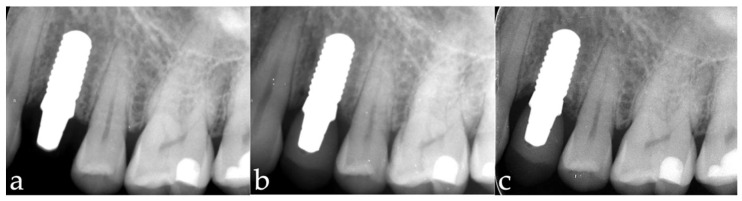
(a–c) Radiographic series: (a) after implantation; (b) crown delivery; (c) 1-year follow-up-up.
